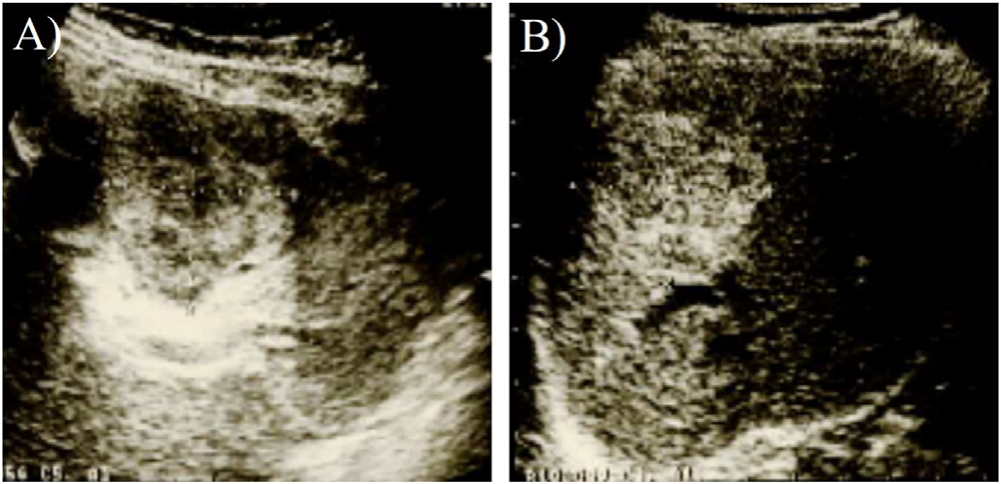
Abdominal ultrasound findings (2001): highly echoic, well-defined lesions measuring 57×51 mm in segment 2/3 of the liver (A) and 41×32 mm in segment 8 of the liver (B) are observed.
